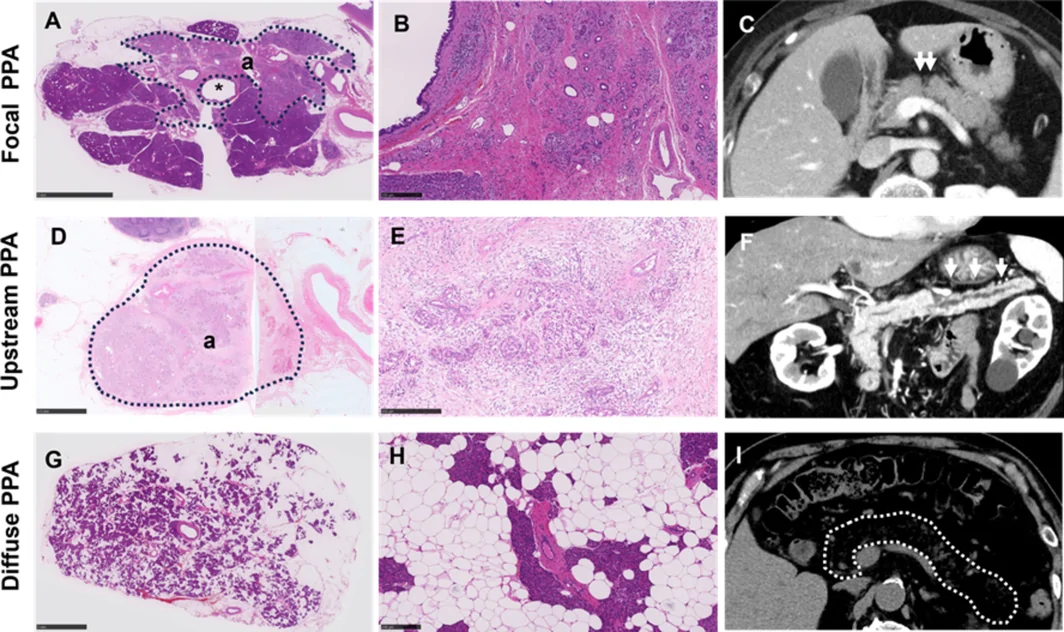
Types of pancreatic parenchymal atrophy (PPA). (A, B) Focal segmental PPA associated with high‐grade pancreatic intraepithelial neoplasia (A, loupe image; B, high‐magnification image). Neoplastic pancreatic ducts, including the main pancreatic duct (asterisk), are mildly dilated. The atrophic area (a) is outlined with a dashed line. (C) Contrast‐enhanced computed tomography (axial image) demonstrating focal segmental PPA corresponding to the pathological findings in (A) and (B). (D, E) Focal global PPA due to upstream obstruction of the main pancreatic duct (D, loupe image; E, high‐magnification image). (F) Contrast‐enhanced computed tomography (coronal image) demonstrating focal global PPA corresponding to the pathological findings in (D) and (E). (G, H) Diffuse PPA associated with marked fat replacement (G, loupe image; H, high‐magnification image). (I) Contrast‐enhanced computed tomography (axial image) demonstrating diffuse PPA corresponding to the pathological findings in (G) and (H). Dashed line indicates the pancreas. Bar = 5 mm (A, G), 2.5 mm (D), and 250 μm (B, E, H).
